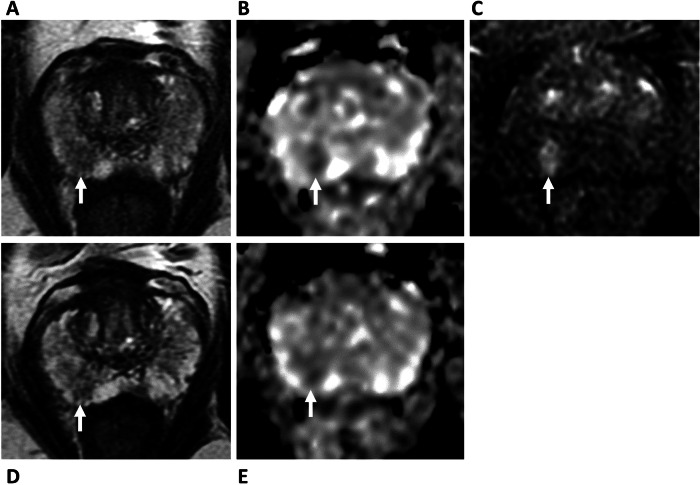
bpMRI versus mpMRI affecting PI-RADS score. 66-year-old patient, presenting PSA 5.88 ng/mL. A–C Baseline MRI shows ill-defined PI-RADS 3 change on T2 (A), with mild restricted diffusion on ADC (B) and *b*-value imaging (not shown), PI-RADS 3, with associated marked focal early enhancement, DCE positive (C, arrow). Overall PI-RADS score 3 + 1 = 4; targeted biopsy shows Gleason 3 + 4 = 7 (Pattern 4 = < 5%) extending for a maximum length of 4.2 mm. D, E MRI at 12 months, PSA 5.43 ng/mL. Stable appearances on Ts (D) and ADC maps (E, arrow); however, PI-RADS score 3, reduced from score 4 due to employment of a bpMRI only
